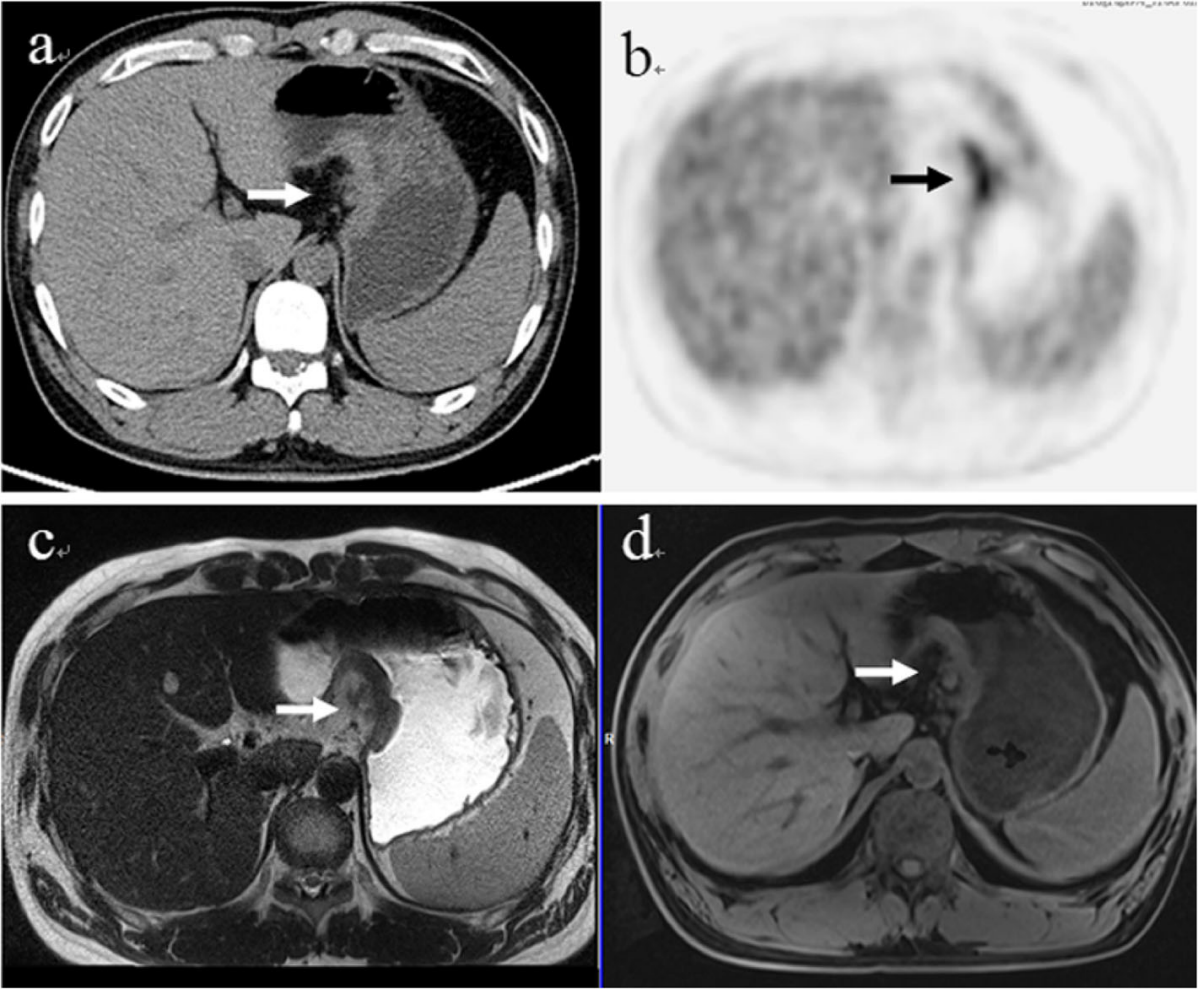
A 34-year-old man with gastric carcinoma at the lesser curvature of the stomach. A nodular exudation of the gastric wall (arrow) was visible in PET/CT (a, b) as well as in T2-HASTE (c) and MRI-T1W (d) images, which is the characteristic information of great value for staging
